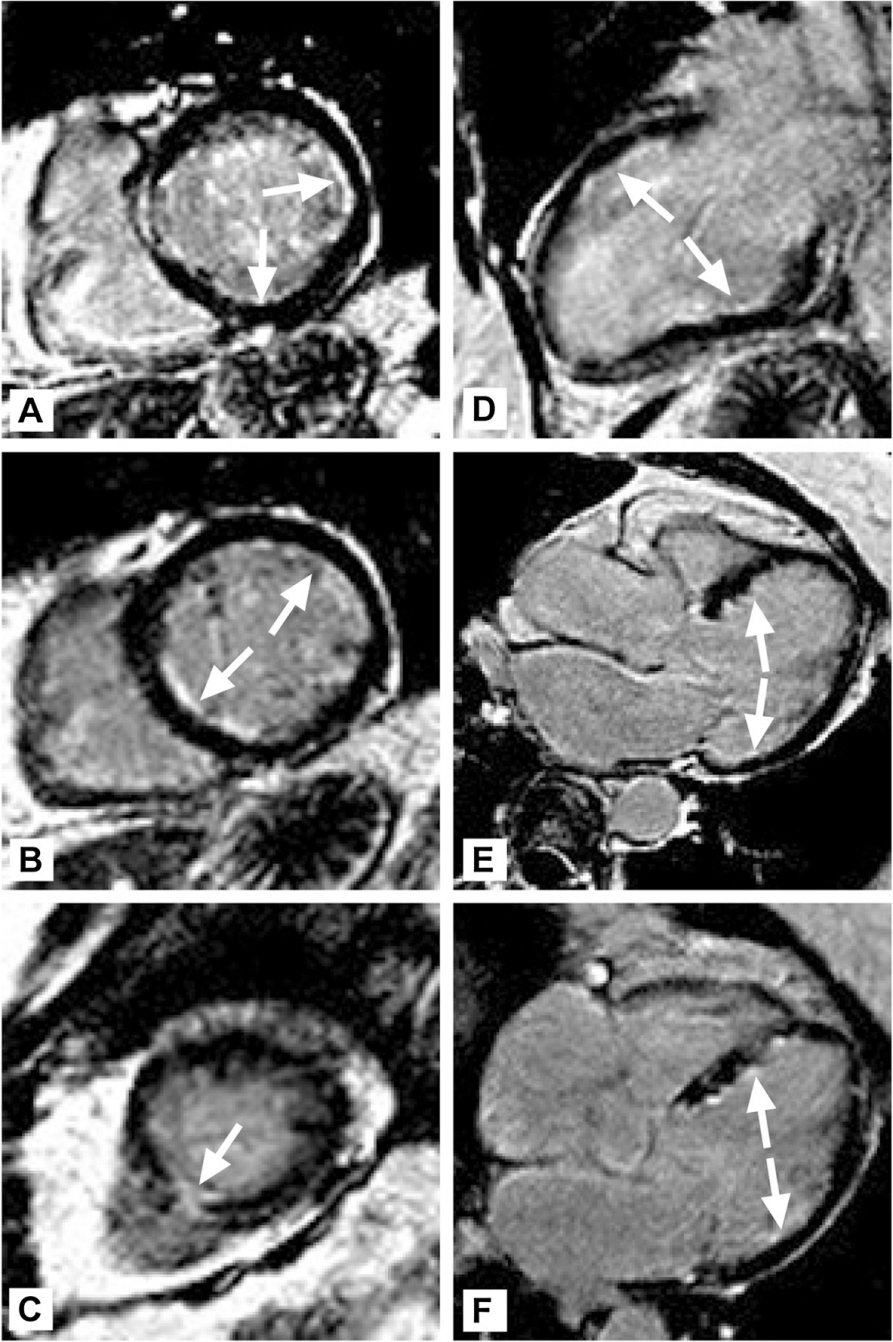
Panels A-F Cardiac Magnetic Resonance Imaging–Phase sensitive inversion recovery gadolinium delayed enhancement images through the left ventricle are shown in short axis planes (A-C): basal, mid and apical cross-sections, and in long axis planes (D-E): 2-chamber view showing the anterior and inferior walls, 3-chamber showing the antero-septal and infero-lateral walls and 4-chamber showing the infero-septal and antero-lateral walls. Normal myocardial signal is nulled and therefore appears black. The white arrows point to the multi-territorial regions of shallow subendocardial hyperenhancement; C–also demonstrates a focus of more transmural inferoapical involvement. Features are in keeping with hypereosinophilic endomyocarditis.
